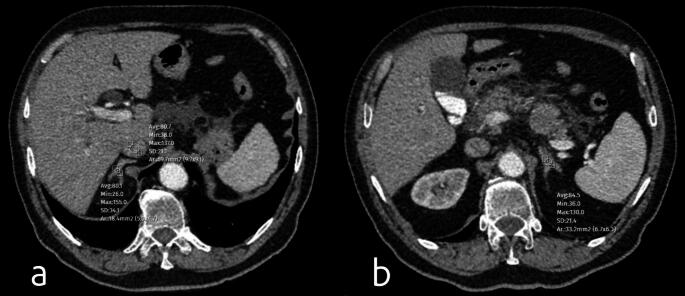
Axial contrast-enhanced CT images demonstrating attenuation measurements of the adrenal glands and inferior vena cava (IVC) in a 56-year-old male patient. The pancreas is enlarged and edematous, with increased density in the peripancreatic fat and localized fluid collections, consistent with Balthazar grade D pancreatitis. The patient did not require ICU admission. (a) Measurement of the right adrenal gland with an average attenuation value of 80.7 HU and the IVC with an average attenuation value of 80.1 HU. (b) Measurement of the left adrenal gland with an average attenuation value of 84.5 HU. Based on these measurements, the adrenal-to-IVC ratio was calculated as 1.03, while the adrenal-to-spleen ratio was 0.62
